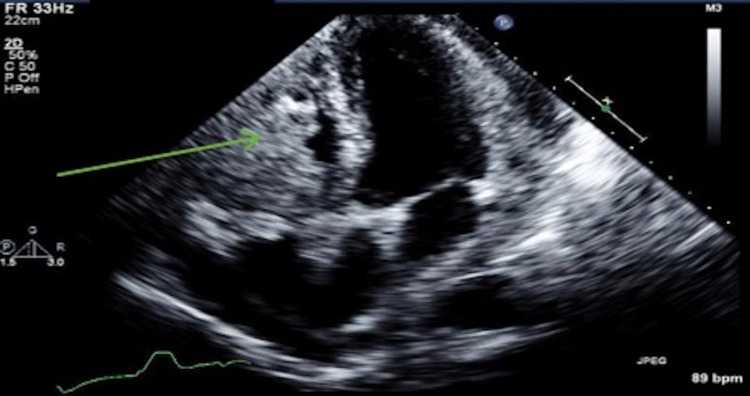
Patient's transthoracic echocardiogram with a large soft-tissue mass (arrow) involving the right ventricle (RV) wall and cavity from the tricuspid annulus to the RV outflow tract. Maximal dimension recorded was 4.6 cm x 3.6 cm.
